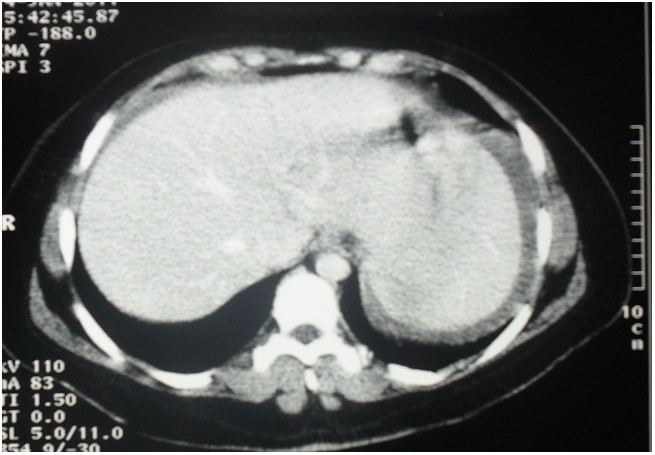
CT scan of the patient with a wandering spleen presenting as a hypogastric mass: absence of the spleen in the left upper quadrant of the abdomen
